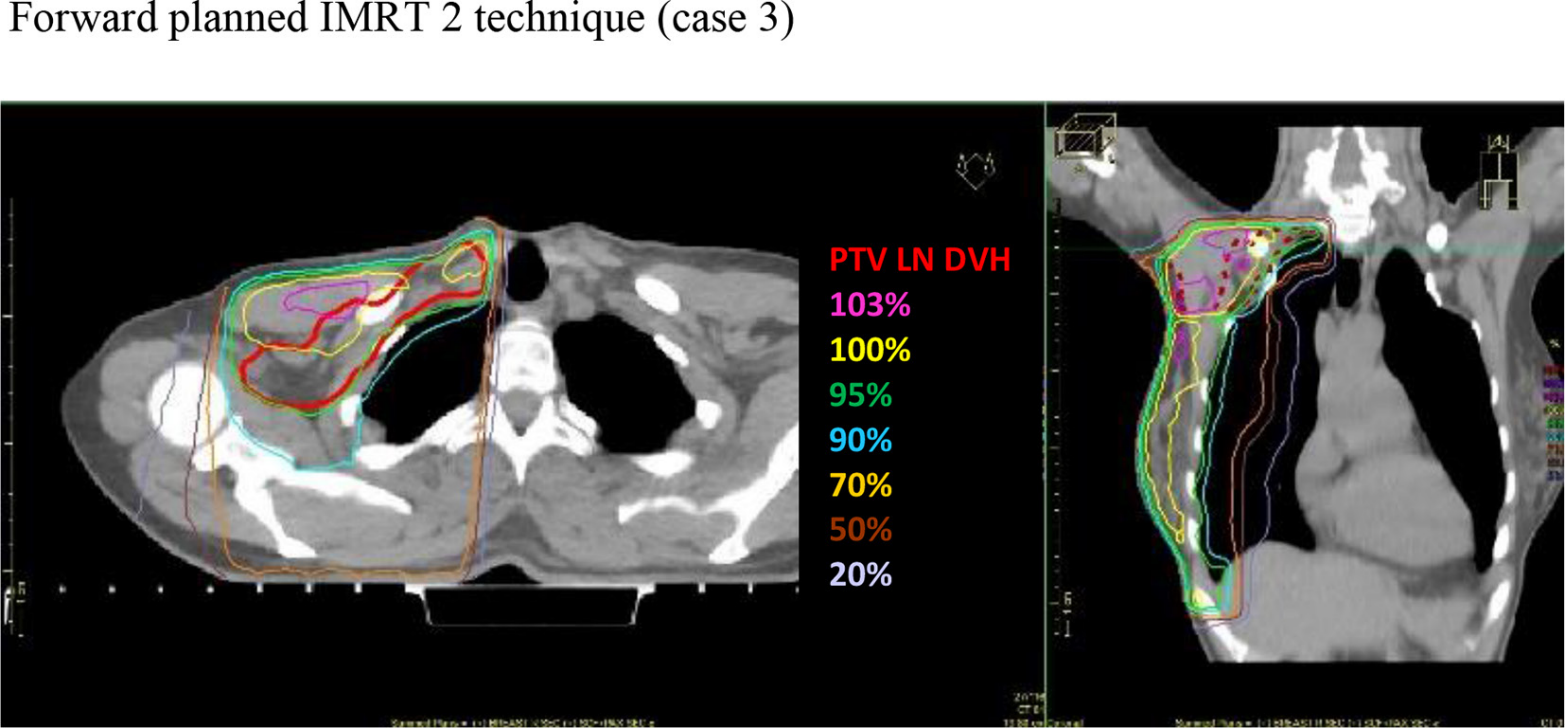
Axial and coronal CT slice views, demonstrating the iso-dose
